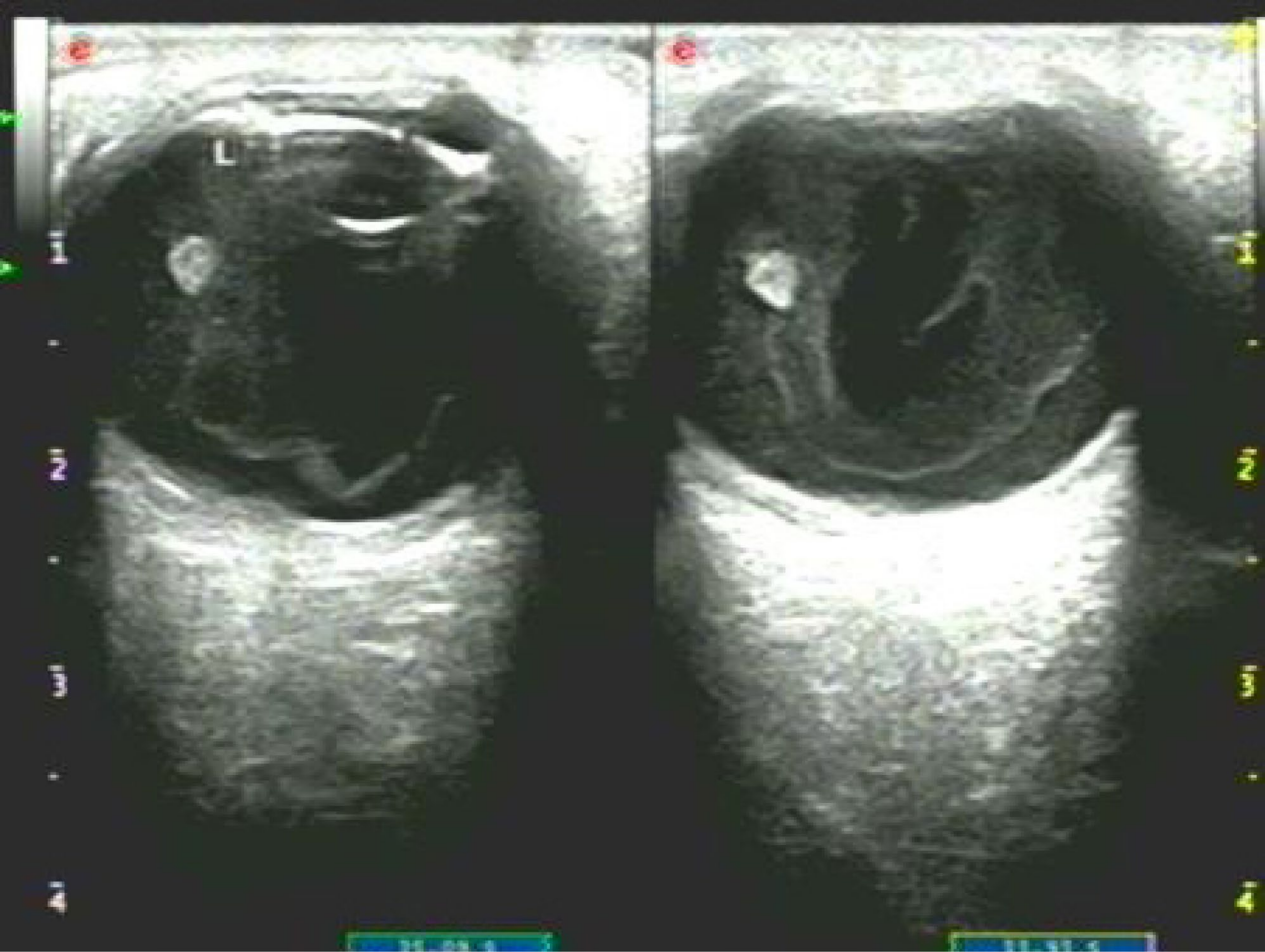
Color Doppler ultrasonography (CDU) of the left eye demonstrating vitreous opacities (medium-to-low reflective echoes)
